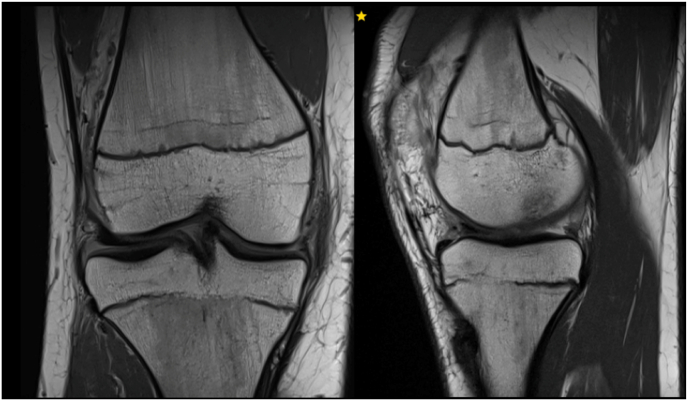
MRI scan showing physeal bar formation in the tibia.
